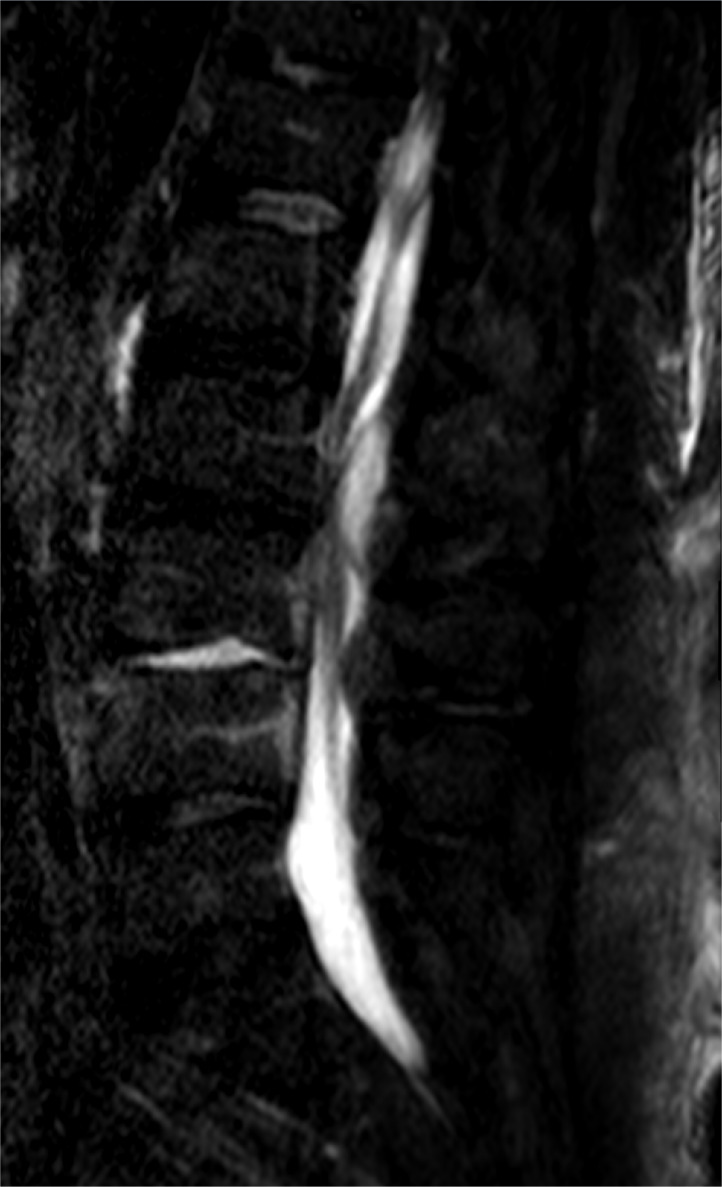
Sagittal STIR MR image of the lumbar spine showing an abnormal
